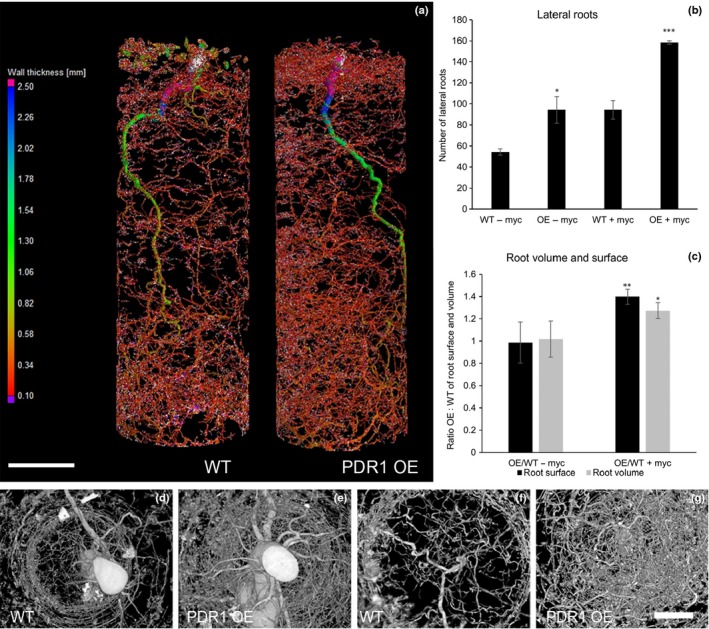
X‐ray computed tomography on wild‐type (WT) and PDR1 OE
